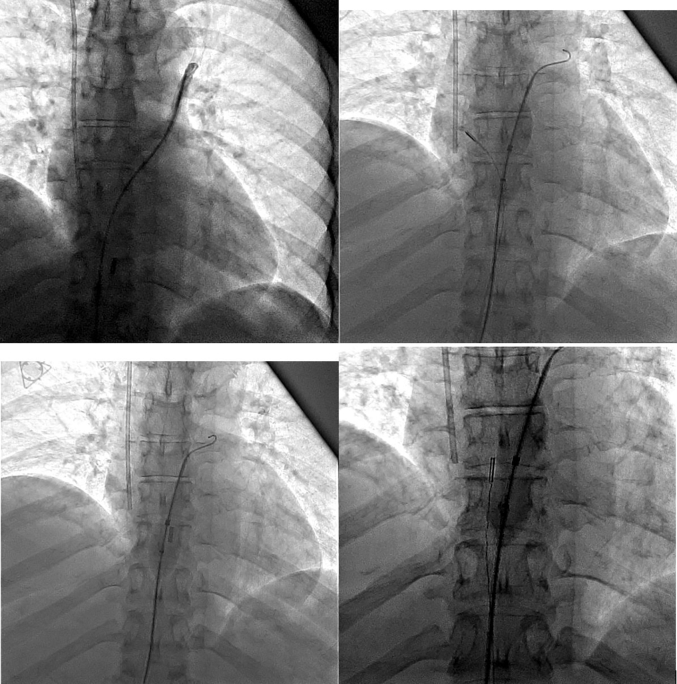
Intracardiac Echocardiography–Guided Right Heart Catheterization With Balloon Sizing of the Patent Foramen Ovale A 24-mm Amplatzer Sizing Balloon was inflated across the interatrial septum to occlude the defect and assess improvement in arterial oxygenation.
